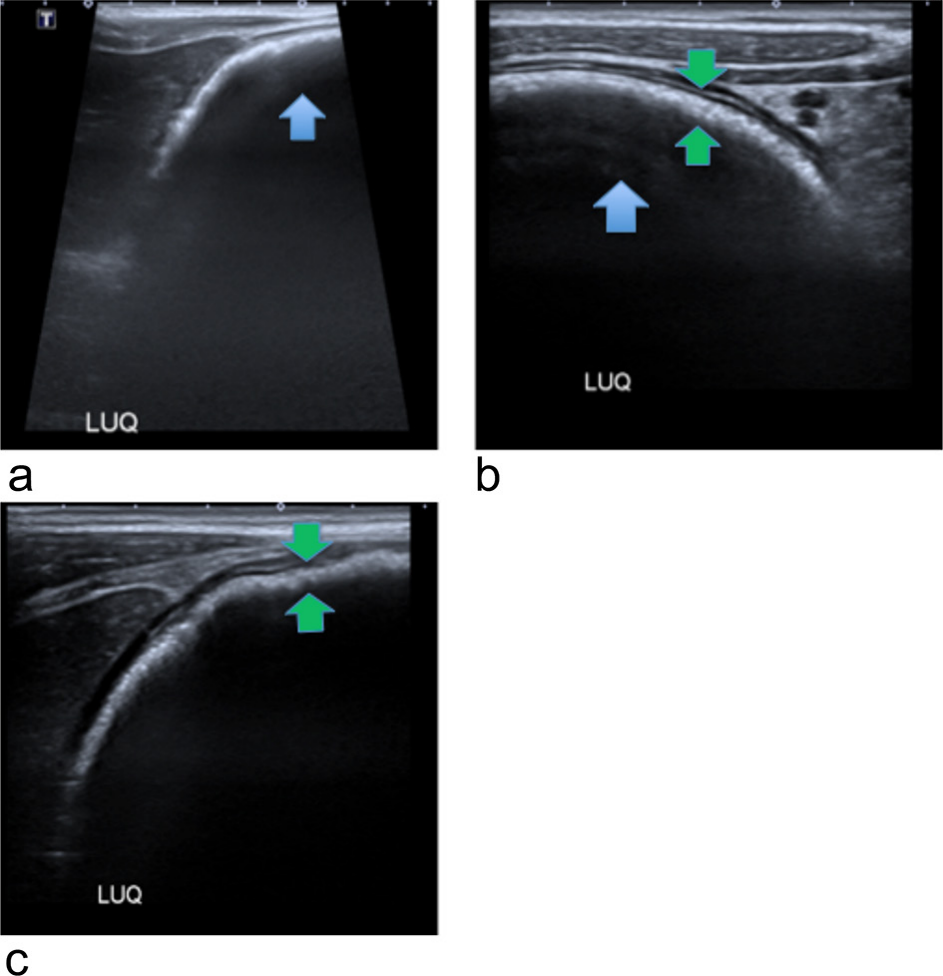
Abdominal ultrasound on 1 February 2018: demonstrating an echogenic curved band (green arrows) with posterior acoustic shadowing (blue arrows) in the left upper quadrant of the abdomen. LUQ, left upper quadrant.
